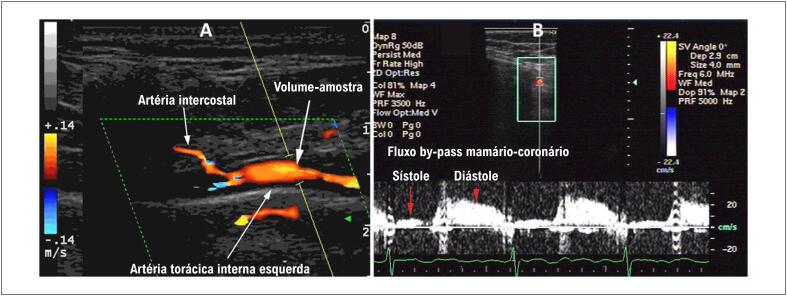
A: detecção do fluxo da artéria torácica interna com posicionamento do volume-amostra do Doppler pulsátil. B: registro do fluxo do enxerto mamário-coronário observando-se fluxo bifásico com predomínio diastólico, indicando que o enxerto se encontra pérvio.
